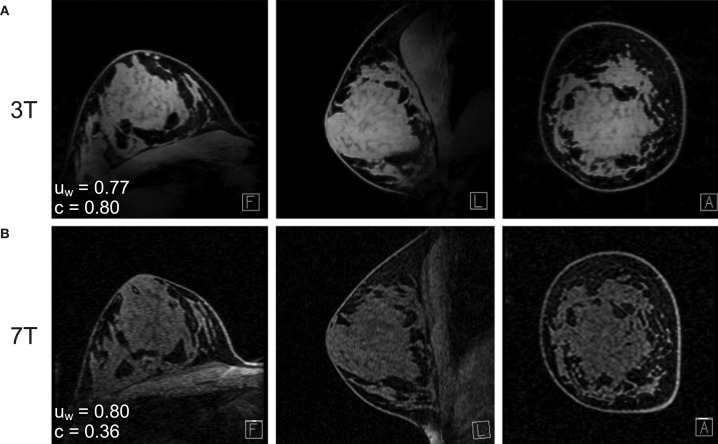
Fat-suppressed T_1_-weighted MRI of the same subject at (A) 7T and (B) 3T. The water signal uniformity (u_w_) is similar across 3T and 7T, while the fat-water contrast (c) is markedly improved at 7T. Reprinted with permission from Brown et al. (28); ©2013 Wiley Periodicals, Inc.
